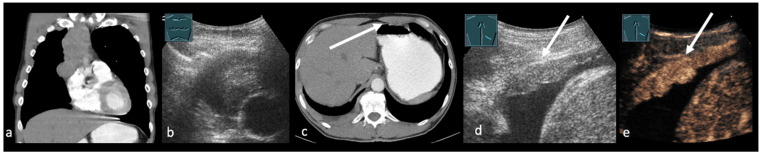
(a–e): Central lung cancer in a CT scan (a). B-US presents a hypoechoic lung tumor in the upper mediastinum (b). In addition, the CT scan shows a suspected pleural metastasis (arrow) (c), which appears as a hyperechoic, nodular structure on B-US (arrow) (d). CEUS (e) shows homogenous contrast enhancement of the lesion (arrow), suggestive of pleural carcinomatosis.
